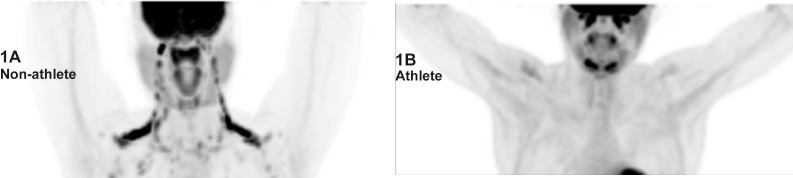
Brown Fat Activity Differences between Athletes and Non-Athletes. (1A) Maximum Intensity Projection of an 18F-FDG PET-CT scan performed in a non-athlete shows multiple and bilateral foci of increased glucose uptake along the laterocervical, supraclavicular, axillary regions, corresponding to metabolically active BAT in these regions. (1B) Maximum Intensity Projection of an 18F-FDG whole body PET-CT scan performed in an athlete. Physiological glucose uptake is seen in the brain, salivary glands and myocardium. No active BAT is seen in this patient.
